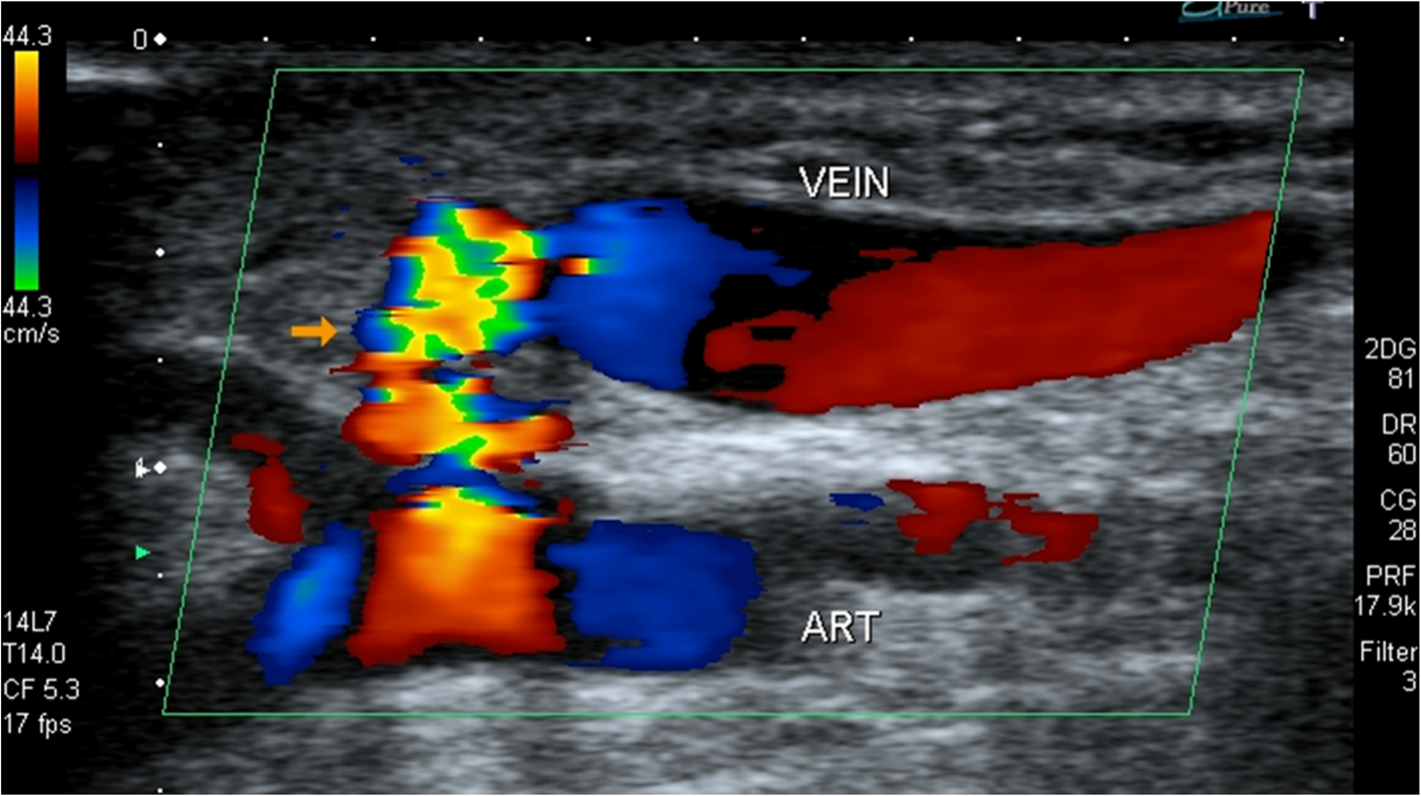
An ultrasound scan of the right radial artery revealed the arteriovenous fistula seen as turbulent high velocity flow (orange arrow) between the vein and the radial artery (ART).
